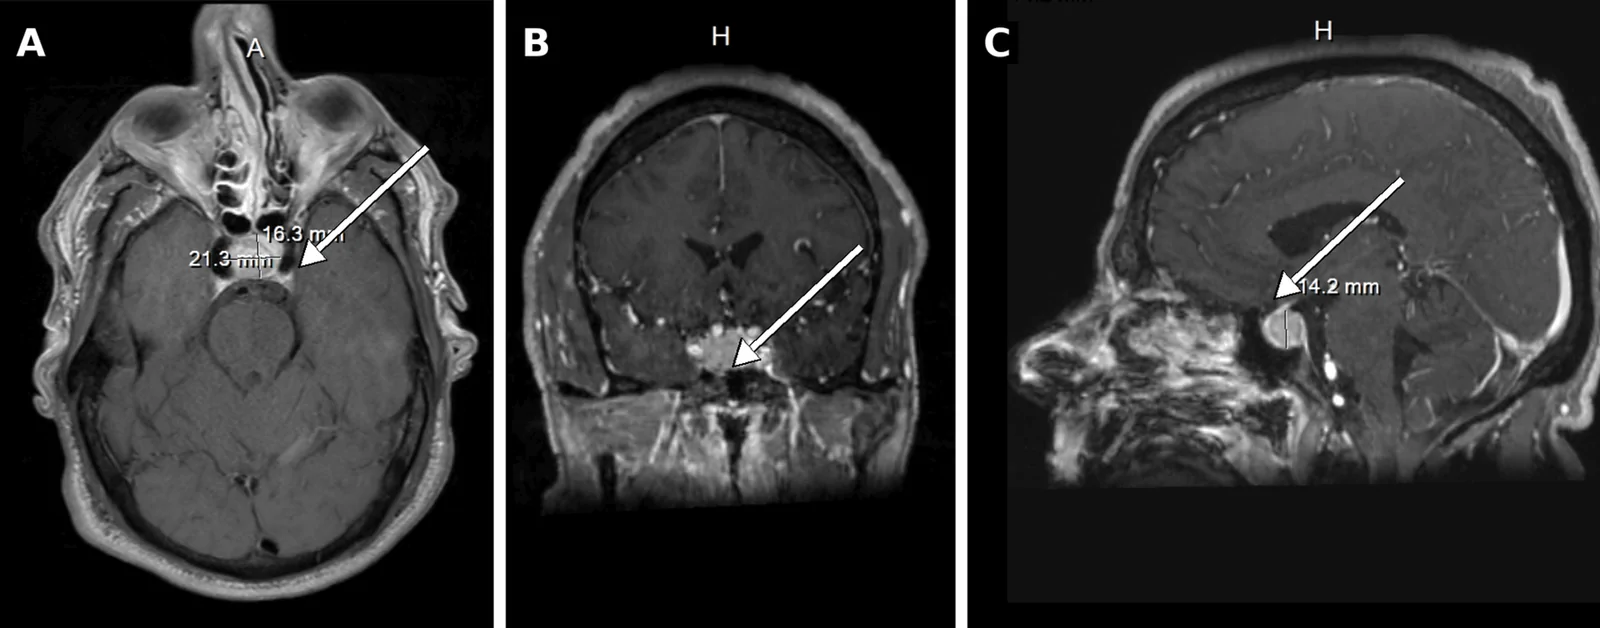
Preoperative Pituitary MRI Immediately Before Surgical Resection, Demonstrating Interval Tumor Growth Preoperative pituitary MRI obtained immediately before surgical resection in March 2025, demonstrating modest interval growth of the macroadenoma over the seven-month delay for cardiac workup. (A) Axial T1-weighted post-contrast image with fat saturation showing the lesion (arrow) with a maximum transverse dimension of 2.1 cm and anteroposterior dimension of 1.6 cm. (B) Coronal T1-weighted post-contrast image with fat saturation showing the enhancing sellar mass (arrow). (C) Sagittal T1-weighted post-contrast image with fat saturation showing the lesion (arrow) with a craniocaudal dimension of 1.4 cm.
